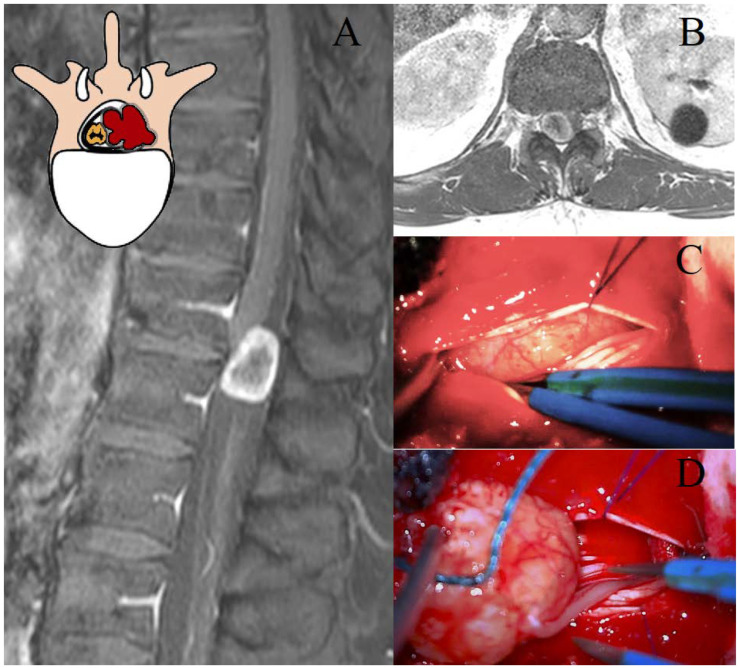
Representation of a T11–T12 thoracic schwannoma, Eden Type I. (A) Preoperative sagittal T1-weighted MRI with contrast, highlighting an intradural-extramedullary lesion at the T11–T12 level, located in the midline and right paramedian region, with peripheral contrast enhancement. (B) Axial scan showing the localization of the lesion. (C) Intraoperative view of dural exposure after laminectomy, with a detail of the lesion. (D) Microsurgical dissection and tumor removal, with identification of the thoracic nerve root of origin, which will be sacrificed.
